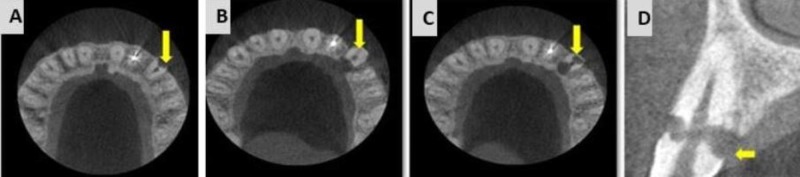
Cone beam computed tomography (CBCT) analysis for tooth 23. (A) CBCT scan: Axial section of tooth 23, showing cervical root resorption, labially. (B) Axial section showing invasive cervical root resorption at cervical level, lingually. (C) Axial section showing invasive cervical root resorption at cervical level, labially and lingually. (D) Sagittal section showing, class III invasive cervical resorption.
